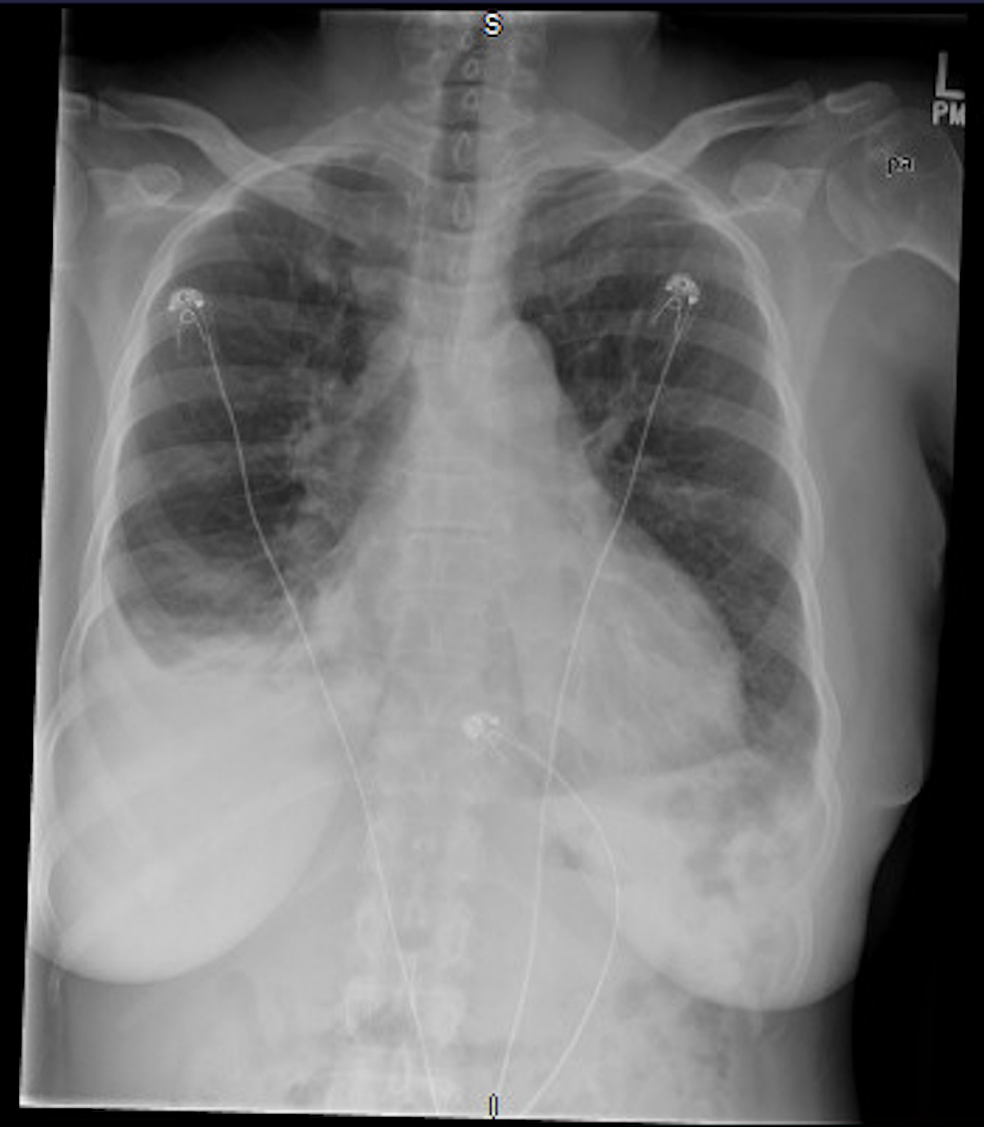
Chest X-ray showing water bottle sign.
